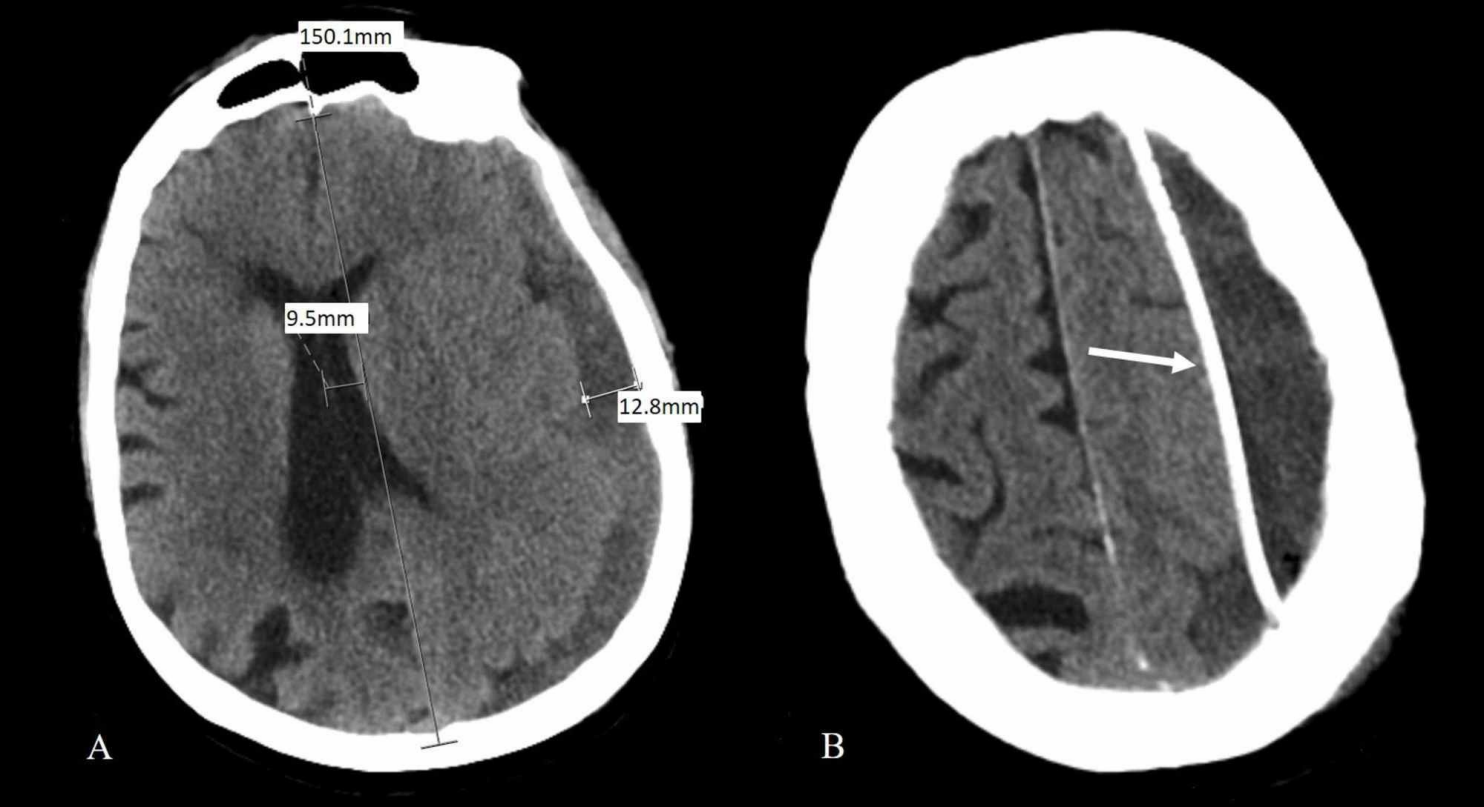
POD 1 CT examination shows the midline shift improved to 9.5 mm with a maximal hematomal depth of 12.8 mm. Residual SDH is relatively more isodense with the brain, suggesting blood is more recent and potentially still coagulated (A). Also note interval catheter placement to the superior aspect of hematoma (B). POD: postoperative day; CT: computed tomography; SDH: subdural hematoma
